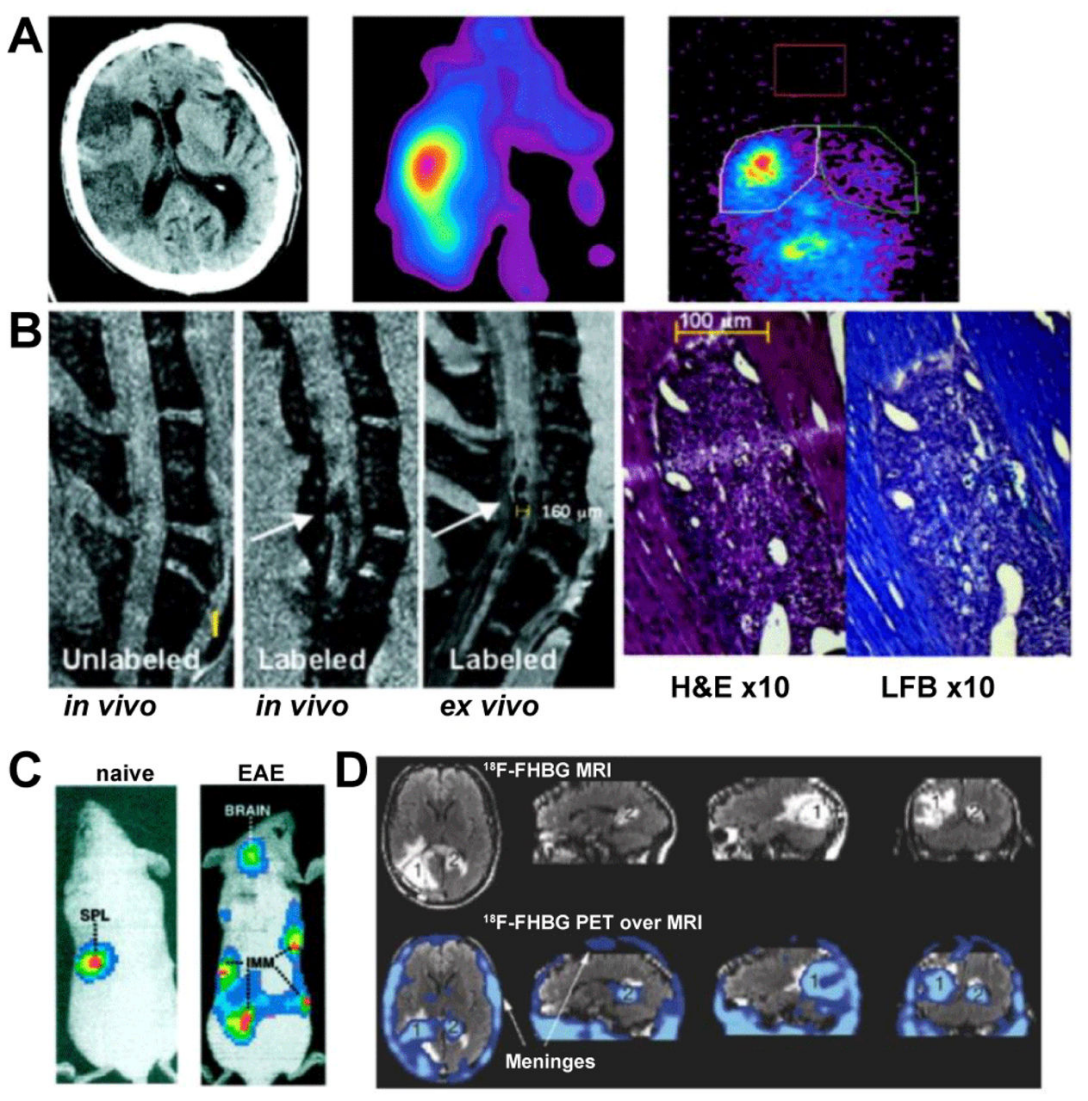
Cell tracking strategies. (A) Noncontrast CT in acute ischemic stroke and SPECT of *in vitro*
